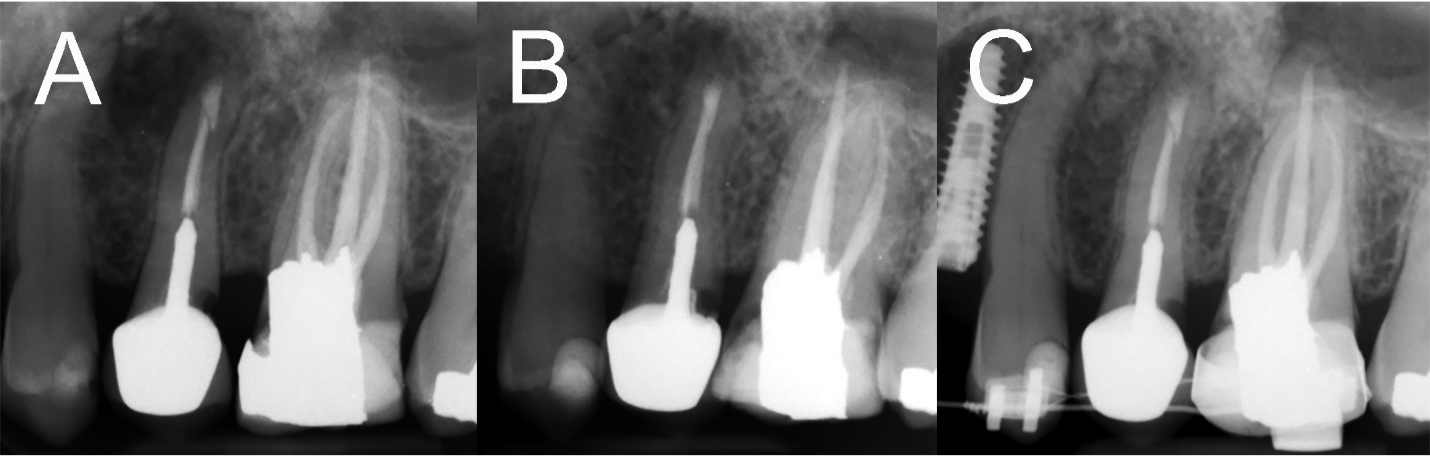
Postoperative radiographic examinations; *A*) Immediately after root-end filling; *B*) 6 months later, healing in progression; *C*) Two-year follow-up, radiographic assessment demonstrated recovery from the lesion and new bone formation
